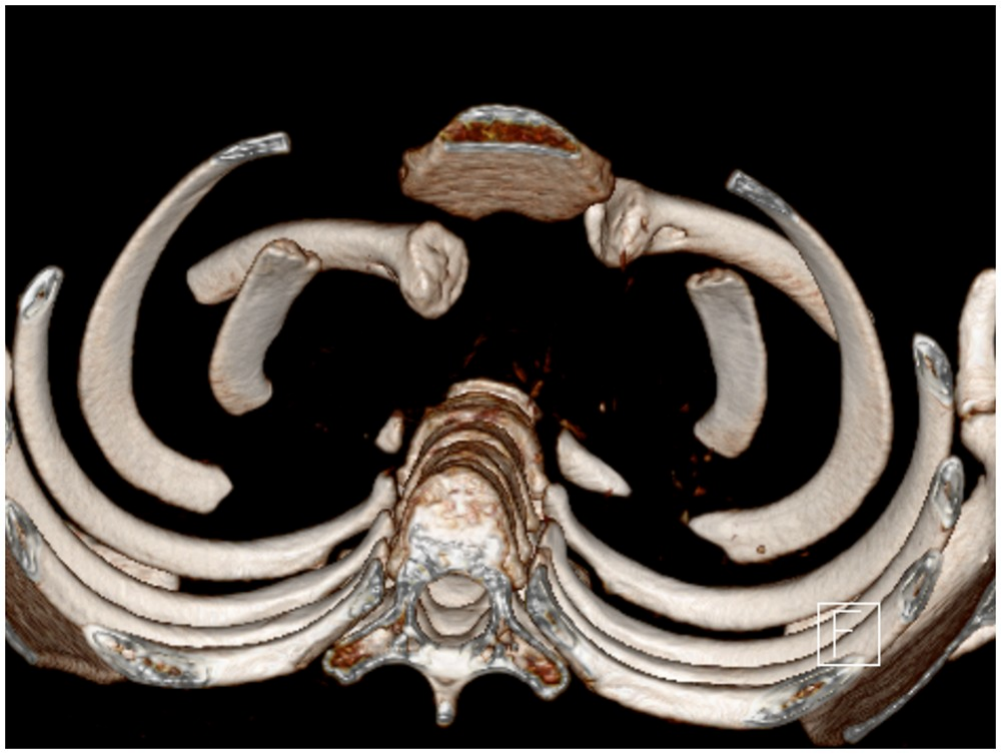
Three-dimensional computed tomography of a posterior sternoclavicular joint (SCJ) dislocation (10-year-old boy, road traffic accident).
